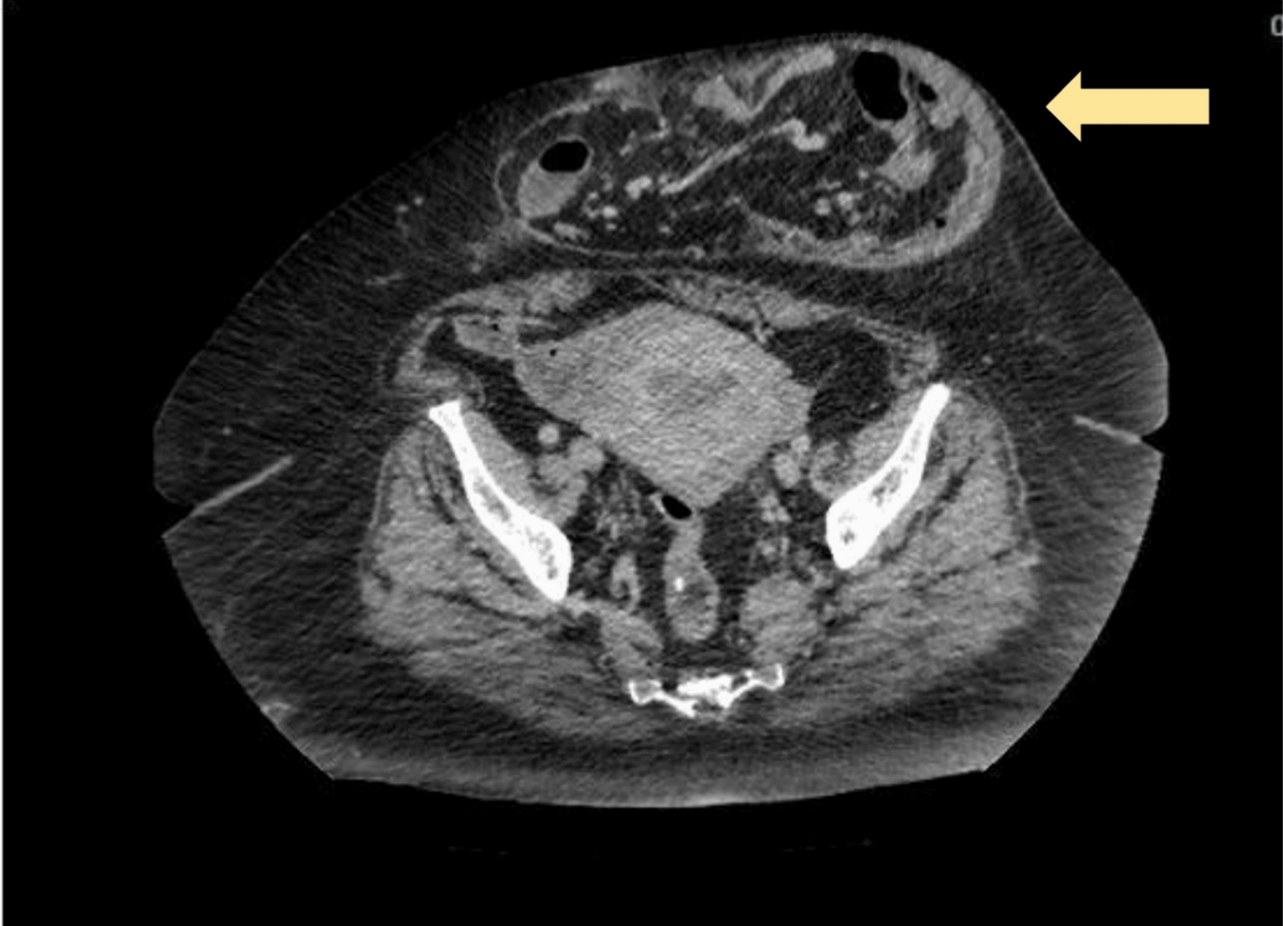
CT abdomen and pelvis (axial view) at the level of the pelvis The image shows the incisional hernia with thickened hernia sac (yellow arrow) CT: computed tomography
